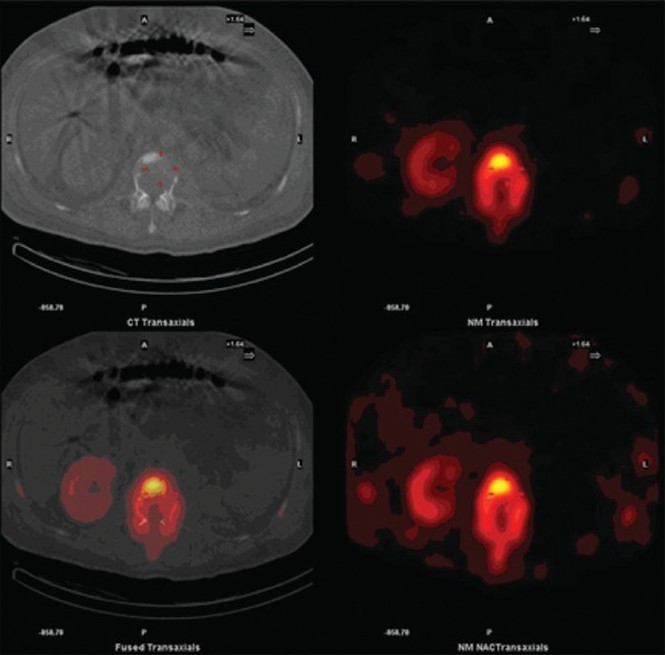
Hybrid SPECT-CT images revealing cortical destruction of the L2 vertebra. The photopenic region in SPECT images corresponding to the site of destruction
